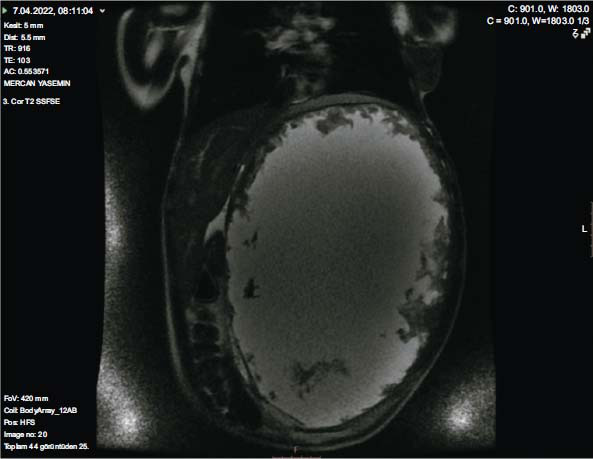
Heterogeneous hyperintense mass with solid (gaucheroma) areas filling the left half of the abdomen in the spleen site on T2-weighted coronal MR imaging.
